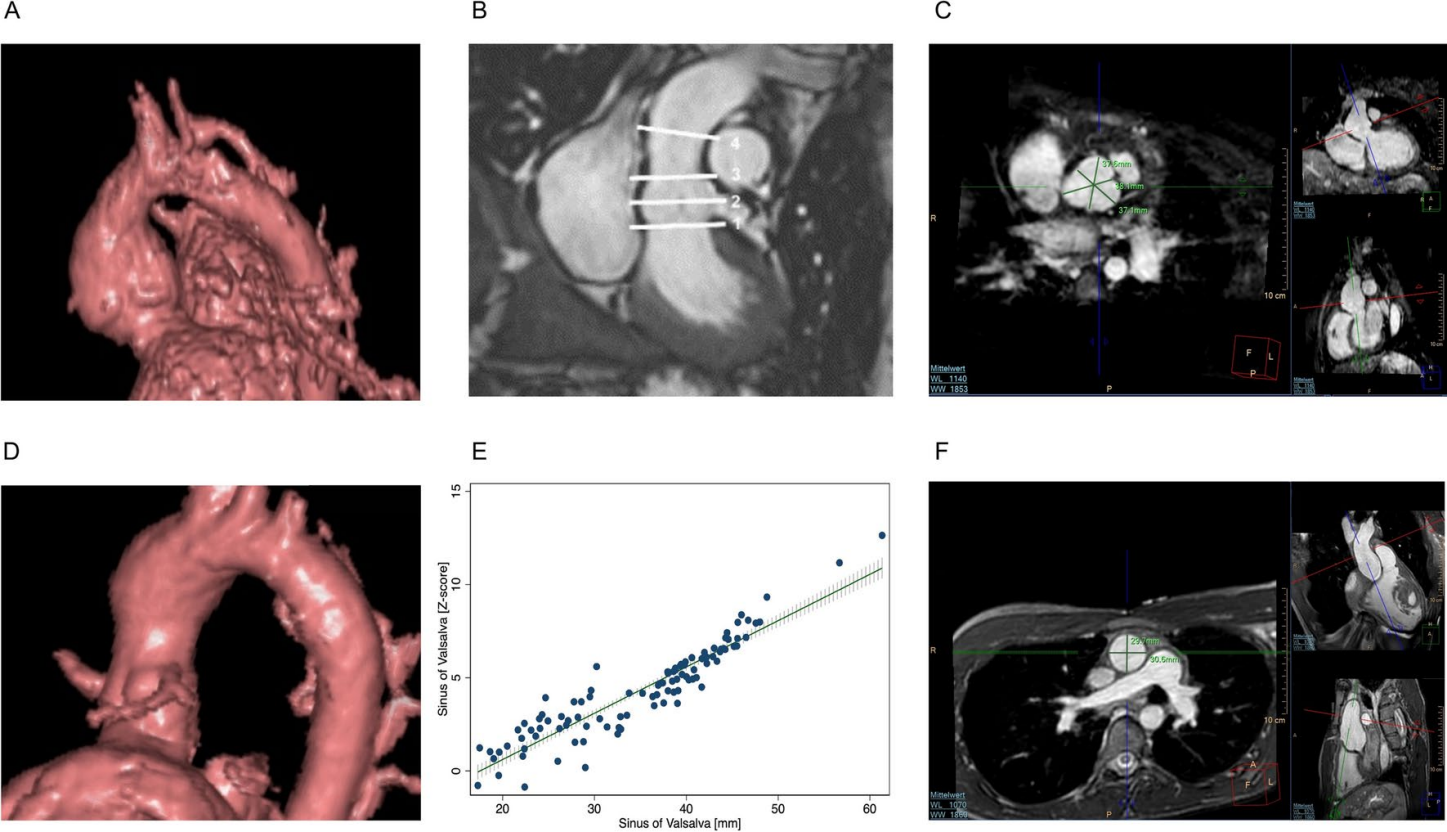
MRI image acquisition *Representative 3D-Reconstruction of autograft after free-root implantation (A) and modified subcoronary implantation (D) created using ViewForum (R6.3V1L7 SP1; Philips Healthcare). Measured locations across the pulmonary autograft (B): 1* = *aortic annulus; 2* = *sinus of Valsalva; 3* = *Sino-tublar junction; 4* = *ascending aorta. Representative 3D whole heart sequence images of sinus of Valsalva (C) and ascending aorta (F). Sinus of Valsalva diameter in relation to sinus of Valsalva diameter normalized to BSA (z-score) (E). MRI* = *Magnetic resonance imaging.*
